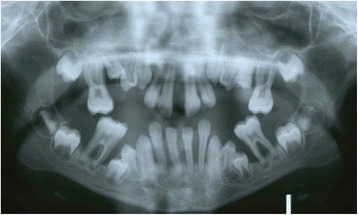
Case 3. Panoramic radiograph showing severe periodontal destruction and migration of teeth
